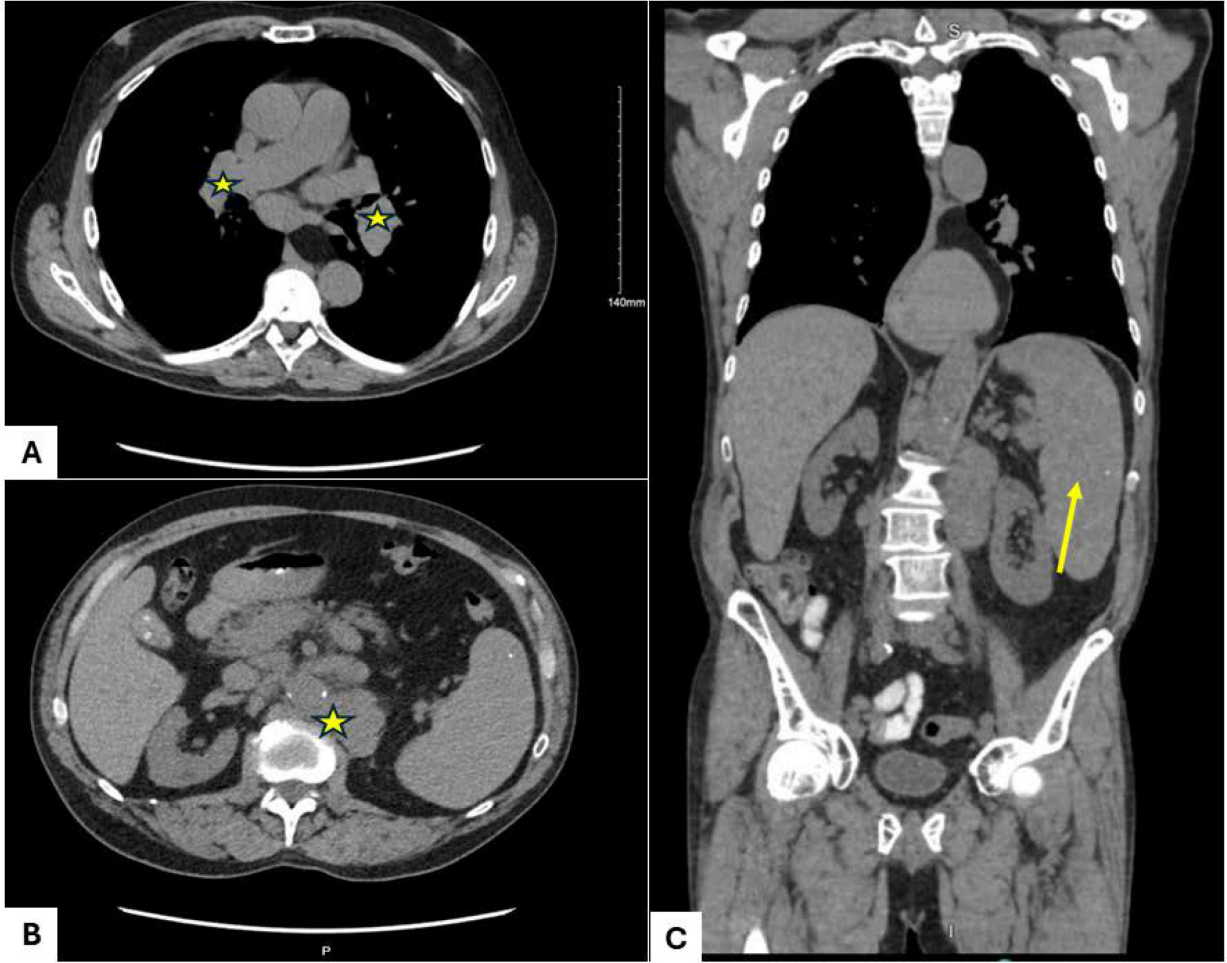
Computed tomography (CT) imaging: (A) Axial view demonstrating enlarged mediastinal lymph nodes (stars). (B) Axial view showing increased subcarinal adenopathy (star). (C) Coronal view demonstrating splenomegaly compared with prior imaging.
